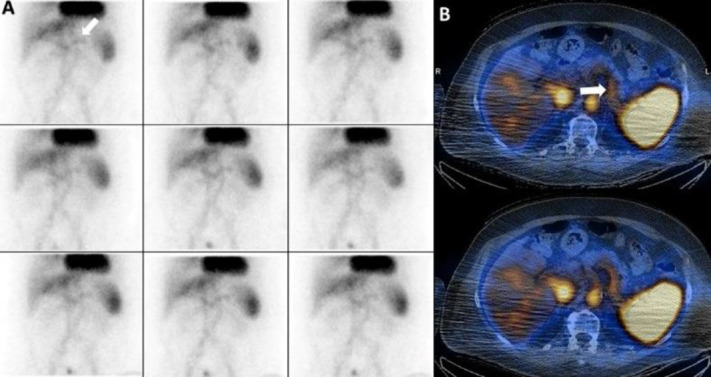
Splenic vessels on planar (A) and SPECT/CT (B)
